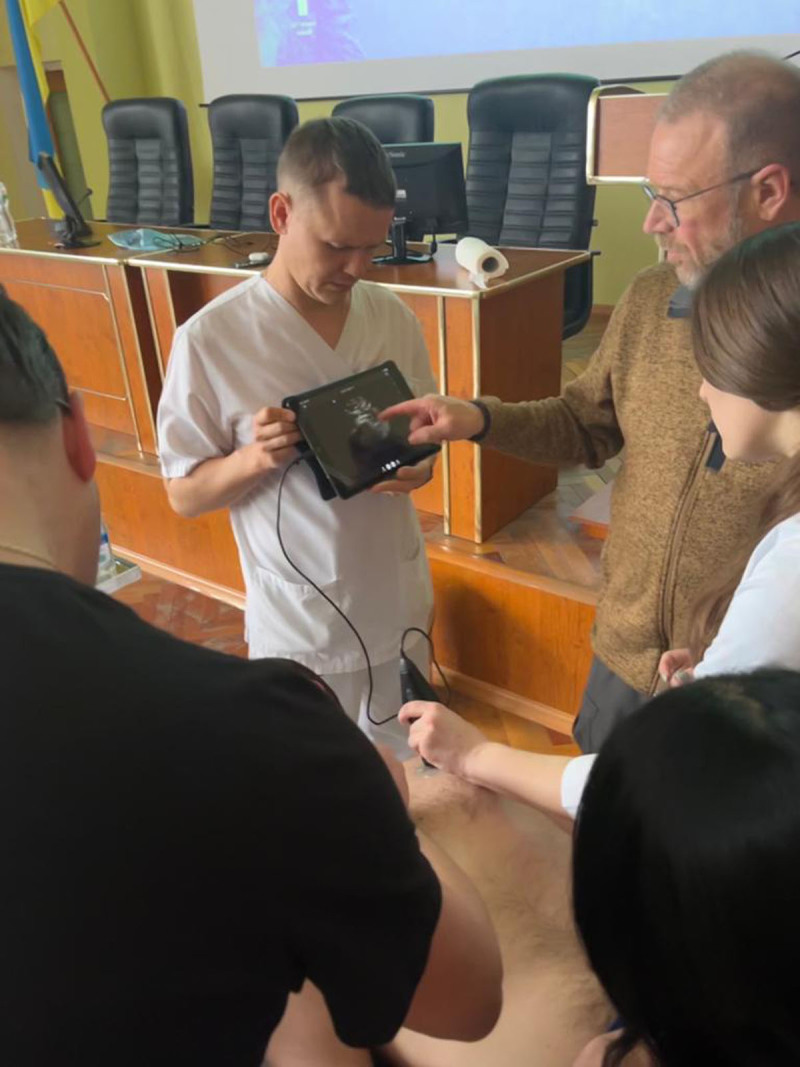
Dr Stevens – brown jacket-instructs student in ultrasound in Kalush City Hospital.
